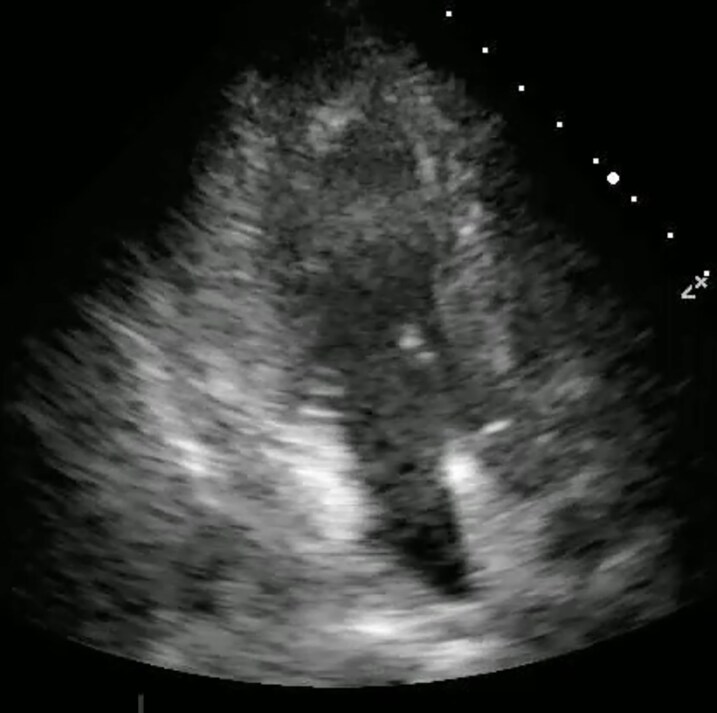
Video_1: transthoracic echocardiography. On admission, transthoracic echocardiography demonstrated moderate pericardial effusion with no distinct regional wall motion abnormalities.
